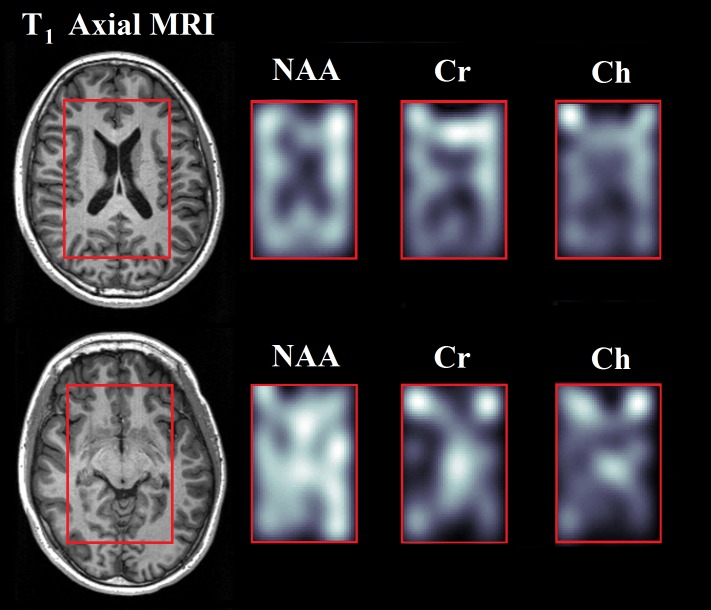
Metabolite maps of NAA, Cr, and Ch from a 16 year old HIV patient are shown. Two T_1_-weighted axial MRI from representative slices are shown on the left. The red box overlaid on the axial images represents the PRESS localization within the slice.
